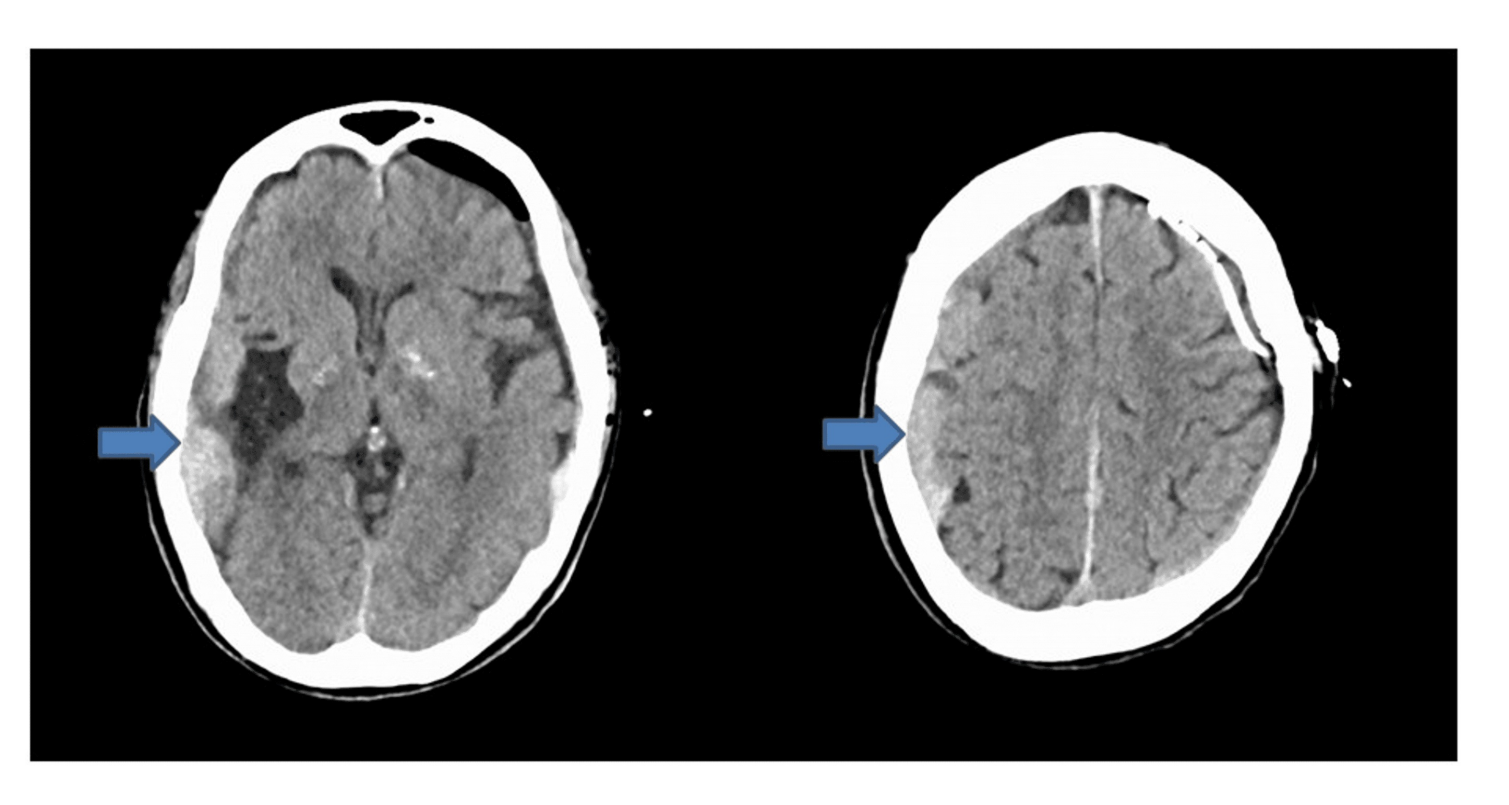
Immediate postoperative CT (six hours and 30 minutes after arrival) following the first surgery, demonstrated complete removal of the left hematoma, no midline shift, and the appearance of a new right acute subdural hematoma (22 mm thick) (arrow).
